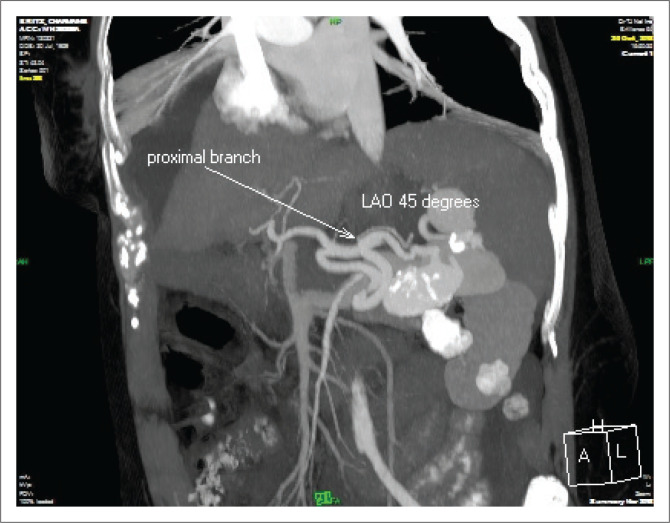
A triphasic liver CT scan displaying a large splenic artery to vein fistula.
